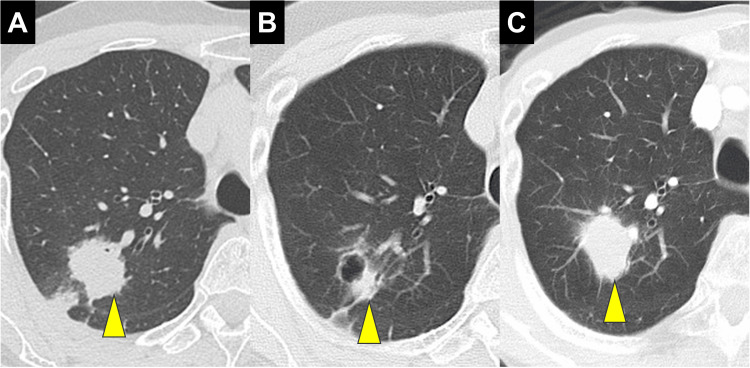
Progression of CT findings during molecular targeted therapy The target nodule is emphasized with arrowheads. (A) A CT scan prior to drug treatment revealed a 27 mm nodule in the right upper lobe. (B) The lung nodule reduced to 12 mm in size after 13 months of afatinib treatment. (C) A subsequent CT scan showed re-progression of the lung lesion to 30 mm 19 months after starting osimertinib.
